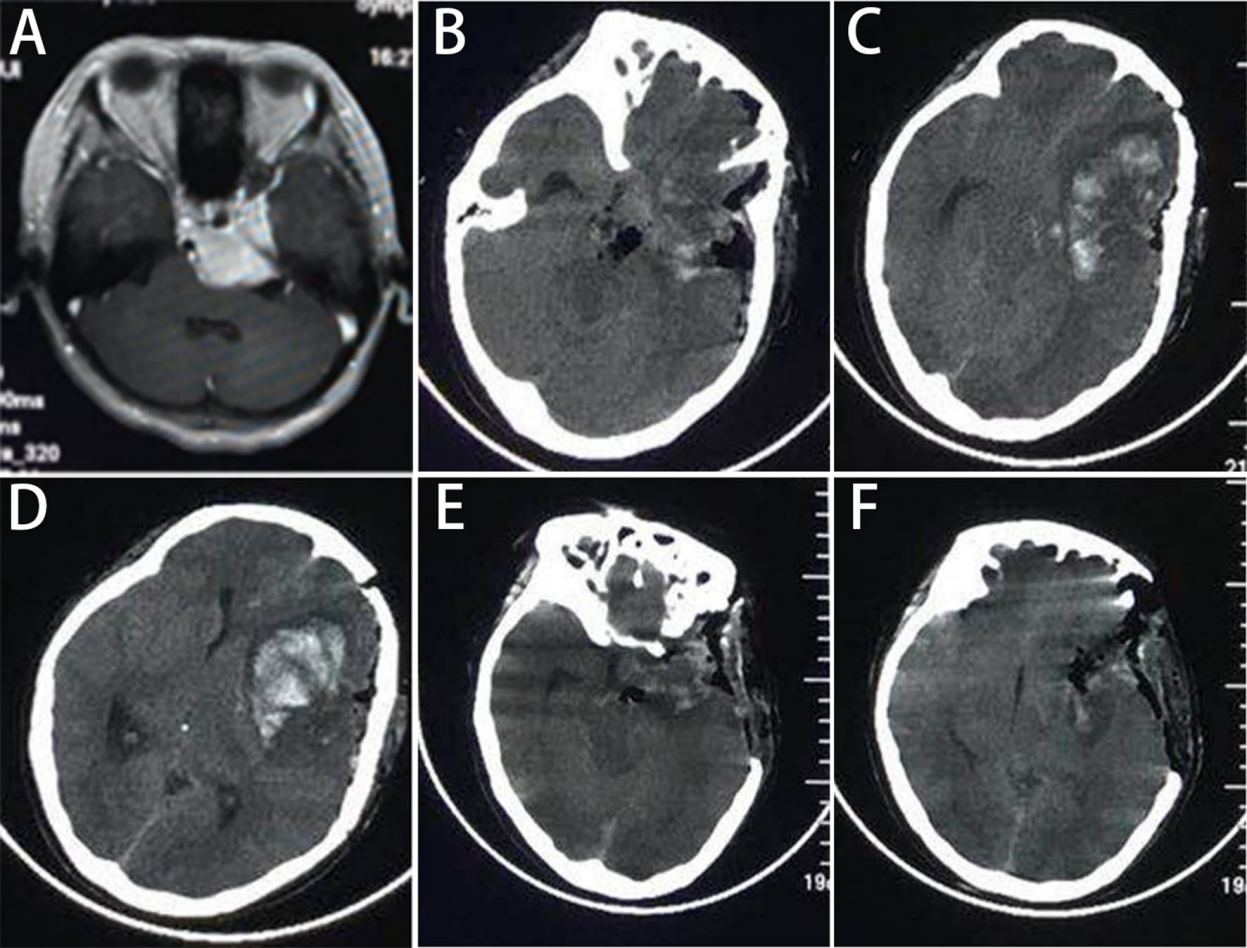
The one patient who had postoperative hematoma and needed second operation. (A) The MR scan shows the cavernous type PCMs, and (B‒D) the CT at 6h postoperatively show a hematoma in the frontal lobe with midline displacement. (E, F) The postoperative CT shows that the hematoma was evacuated.
